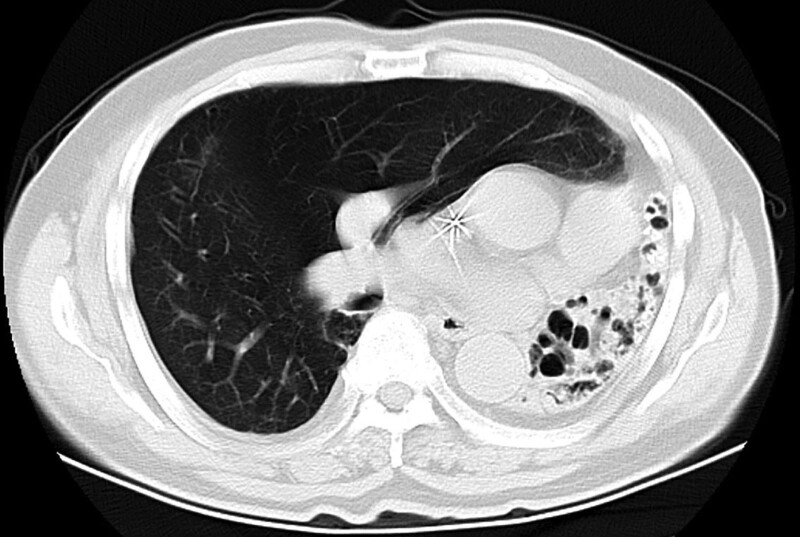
CT scan of the lung window showing the mediastinum and heart shifted to the left with hyperinflation and expansion of the right lung. The left lung is almost entirely hypoplastic with multiple dilated bronchi and a cystic morphology. CT = computed tomography.
